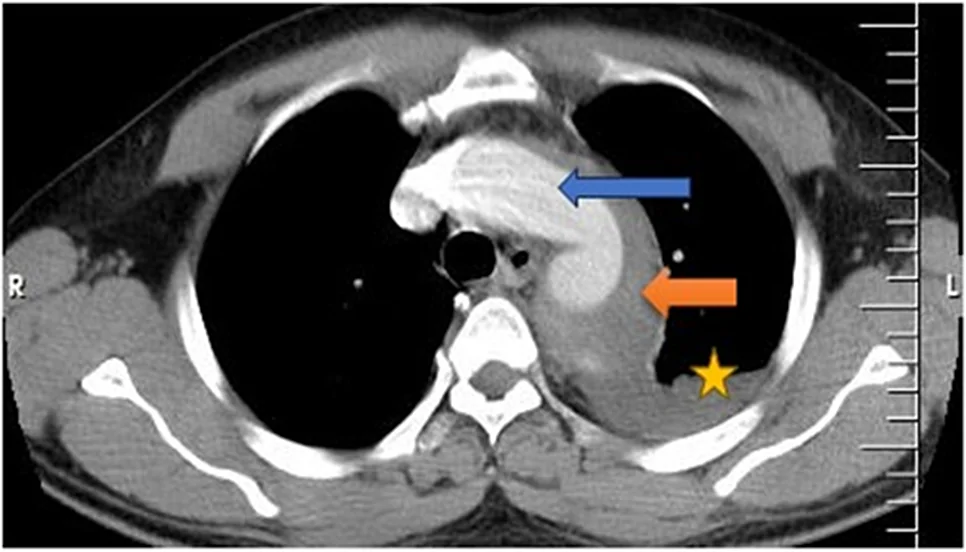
Post-contrast axial chest CT image with significant aortic aneurysm showing the dissected aortic arch. True lumen (blue arrow), false lumen (orange arrow), and a left-sided pleural effusion (star), often a sign of periaortic inflammation or incipient rupture
